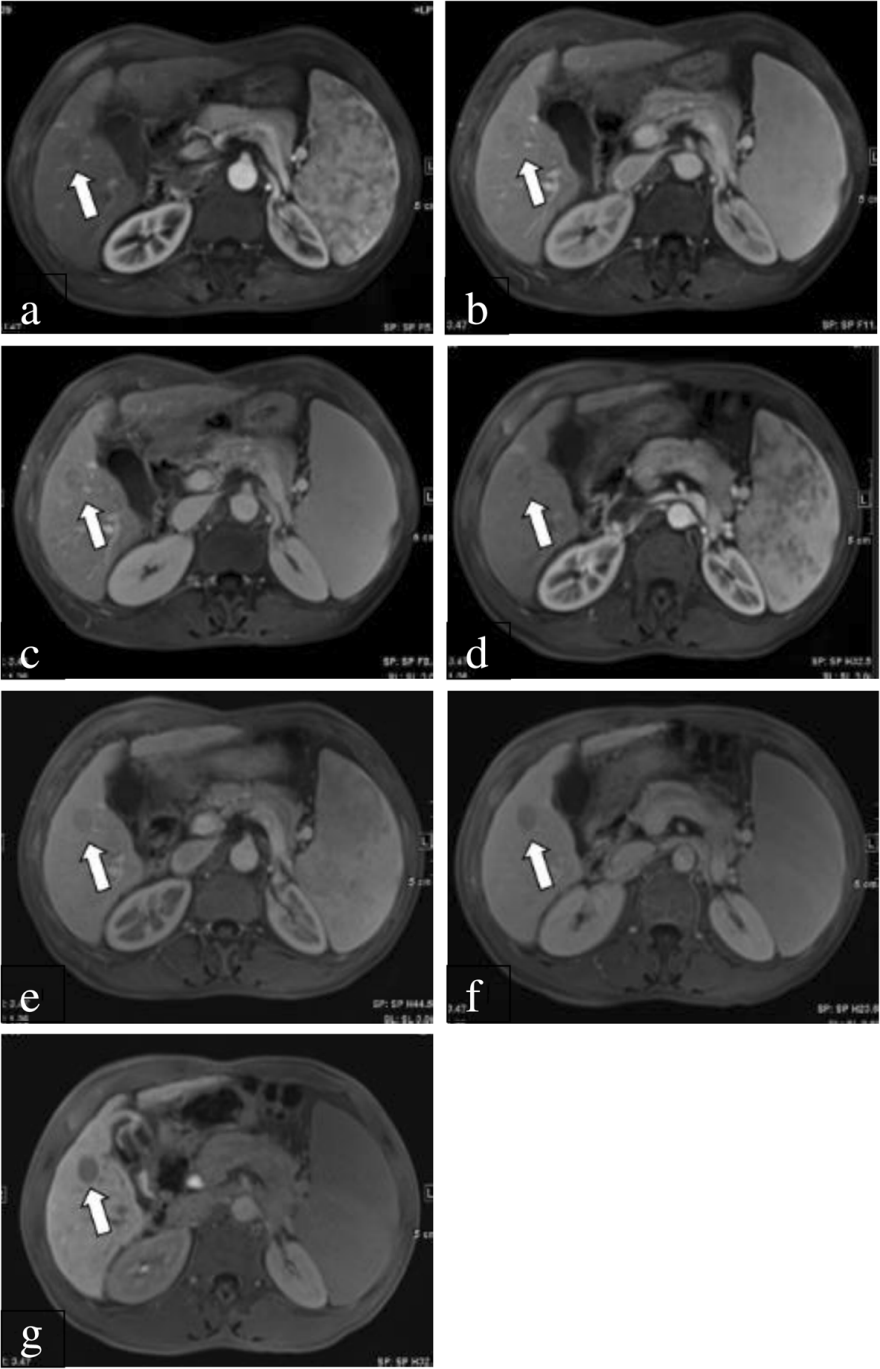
A 63-year-old male patient with HCC confirmed by surgery. a: On gadopentetate dimeglumine-enhanced 1.5-T MRI, there was a 17-mm nodule (arrow) showing arterial enhancement. b: During the PVP, the nodule (arrow) as hypointense (washout) with a capsule appearance. c: During the delayed phase, the nodule (arrow) had a capsule appearance that was visible as a delayed hyperenhancing rim. d: Five days later, the patient underwent gadoxetic acid-enhanced 1.5-T MRI. There was a 17-mm nodule (arrow) showing arterial enhancement. e: During the PVP, the nodule (arrow) was hypointense (washout) without a capsule appearance. f: Additionally, during the delayed phase, the nodule (arrow) was hypointense without a capsule appearance. g: During the HBP, the nodule (arrow) was hypointense without a capsule appearance
